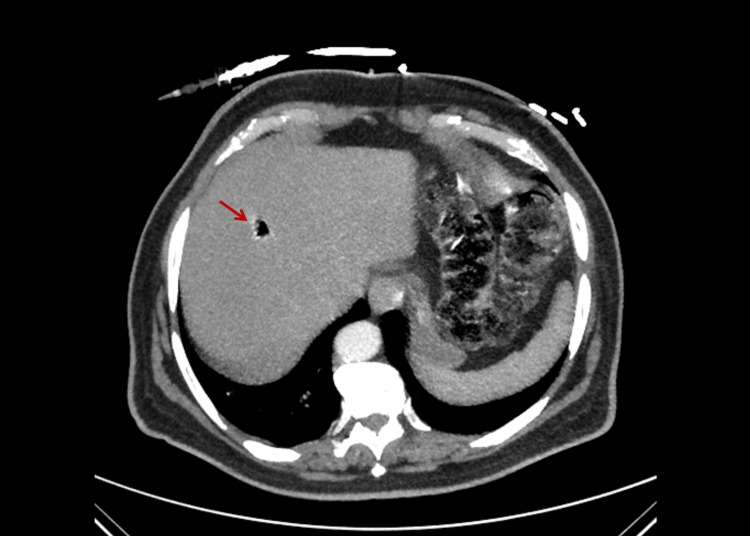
Gas-filled liver abscess on abdominal CT scan. Well circumscribed air-dense lesion with 13 mm in diameter in the right hepatic lobe (arrow), without contrast-enhanced areas in the surrounding parenchyma, association to vascular structures, or pneumobilia. Although initially interpreted as an artifact, the lesion is more suggestive of an abscess caused by a gas-producing pathogen since it was confirmed on additional imaging tests.
